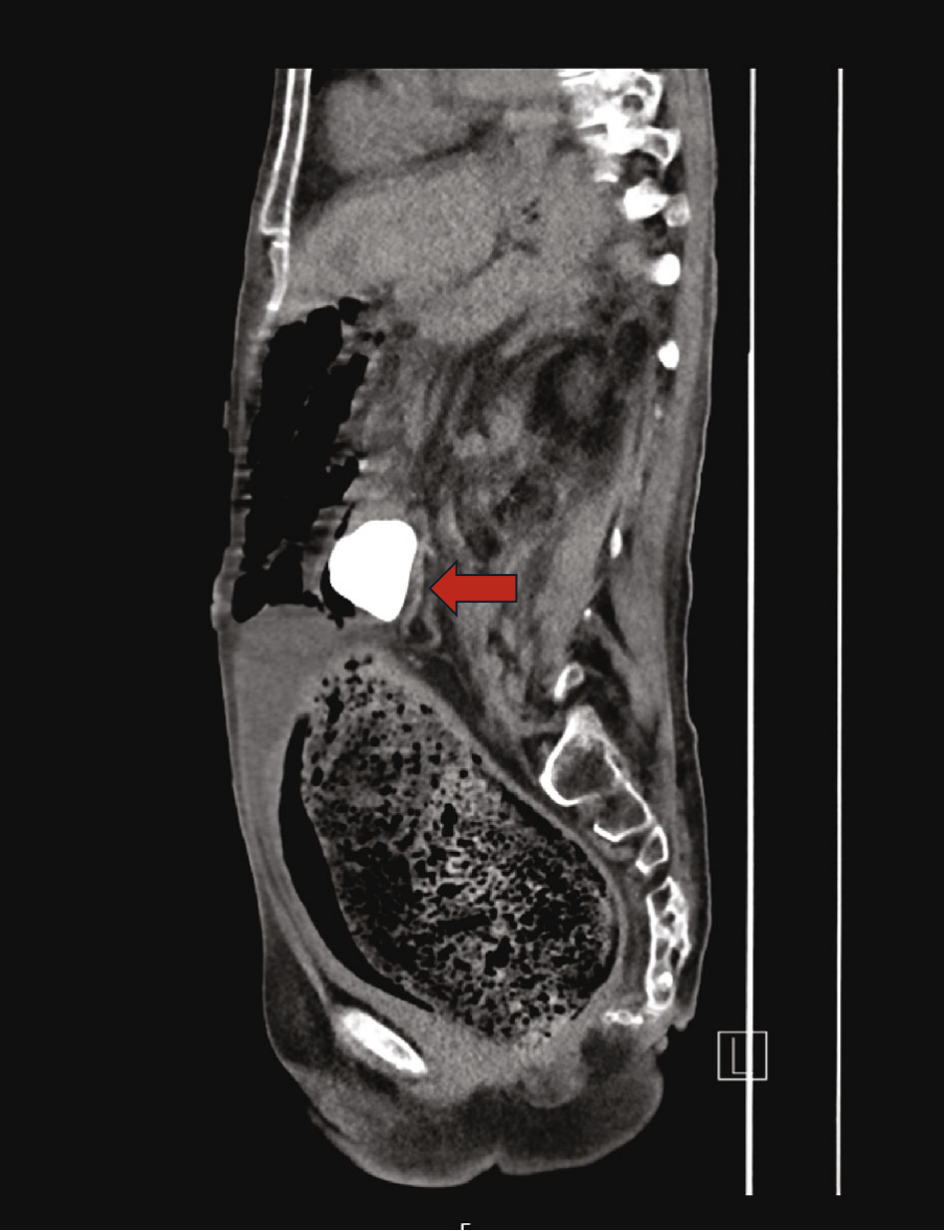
Sagittal plane of the initial CT scan, displaying the staghorn calculus within the renocolic fistula, measuring 3.9 cm.
